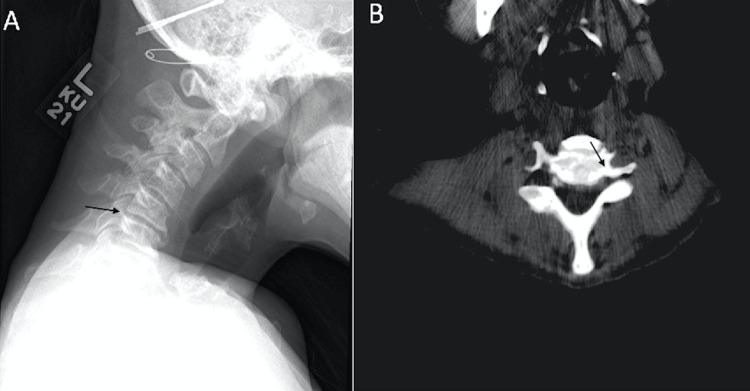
A) Lateral X-ray of the cervical spine. B) Transverse non-contrast cervical CT scan CT: computed tomography
